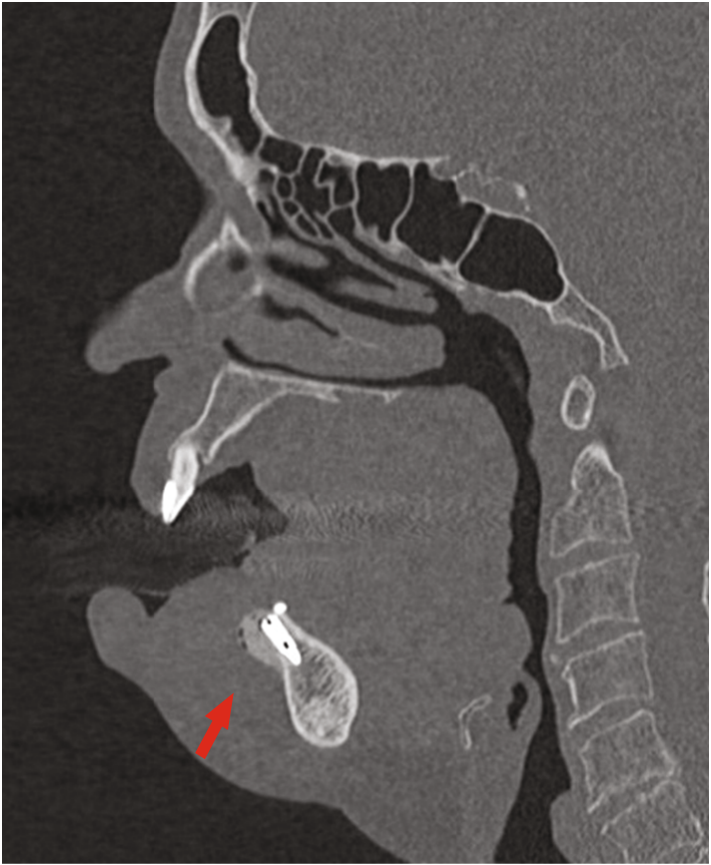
CT sagittal section after removing nasotracheal tube: fixture with the correct axis of insertion without lingual cortical perforation. The buccal hematoma is still present, whereas the tongue is less displaced. The red arrow shows horizontal GBR with bone grafting materials.
